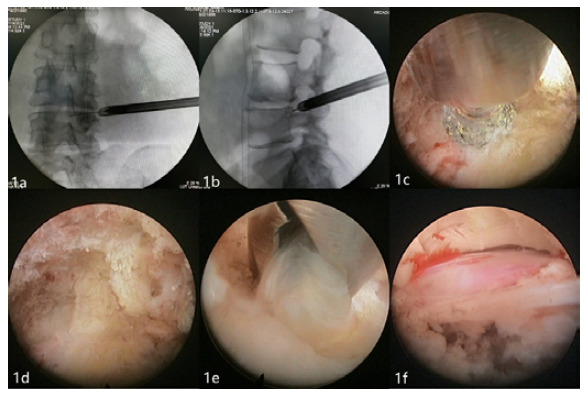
Operation procedure for the full-endoscopic visualization technique via the transforaminal approach. Intraoperative anteroposterior and lateral radiographs confirmed the intervertebral gap and foramina (a, b); The ventral superior articular process was shaped by a power drill (c); Ligamentum flavum and posterior edge of the intervertebral disc (d); Removal of the prominent nucleus pulposus (e); Exploration of nerve root relaxation with no nucleus pulposus left (f).
